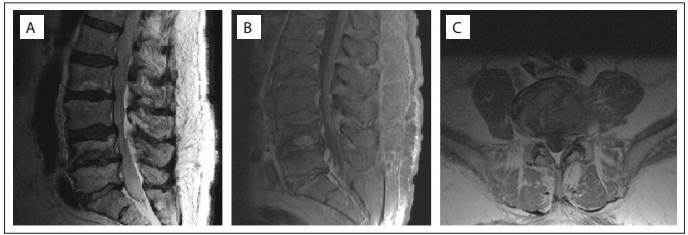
Magnetic resonance imaging (MRI) after 36 months of antibiotic therapy showing reduction of paraspinal soft-tissue edema and abscess. (A) Lateral T2 view. (B) Lateral T1 gadolinium view. (C) Axial T1 gadolinium view.
